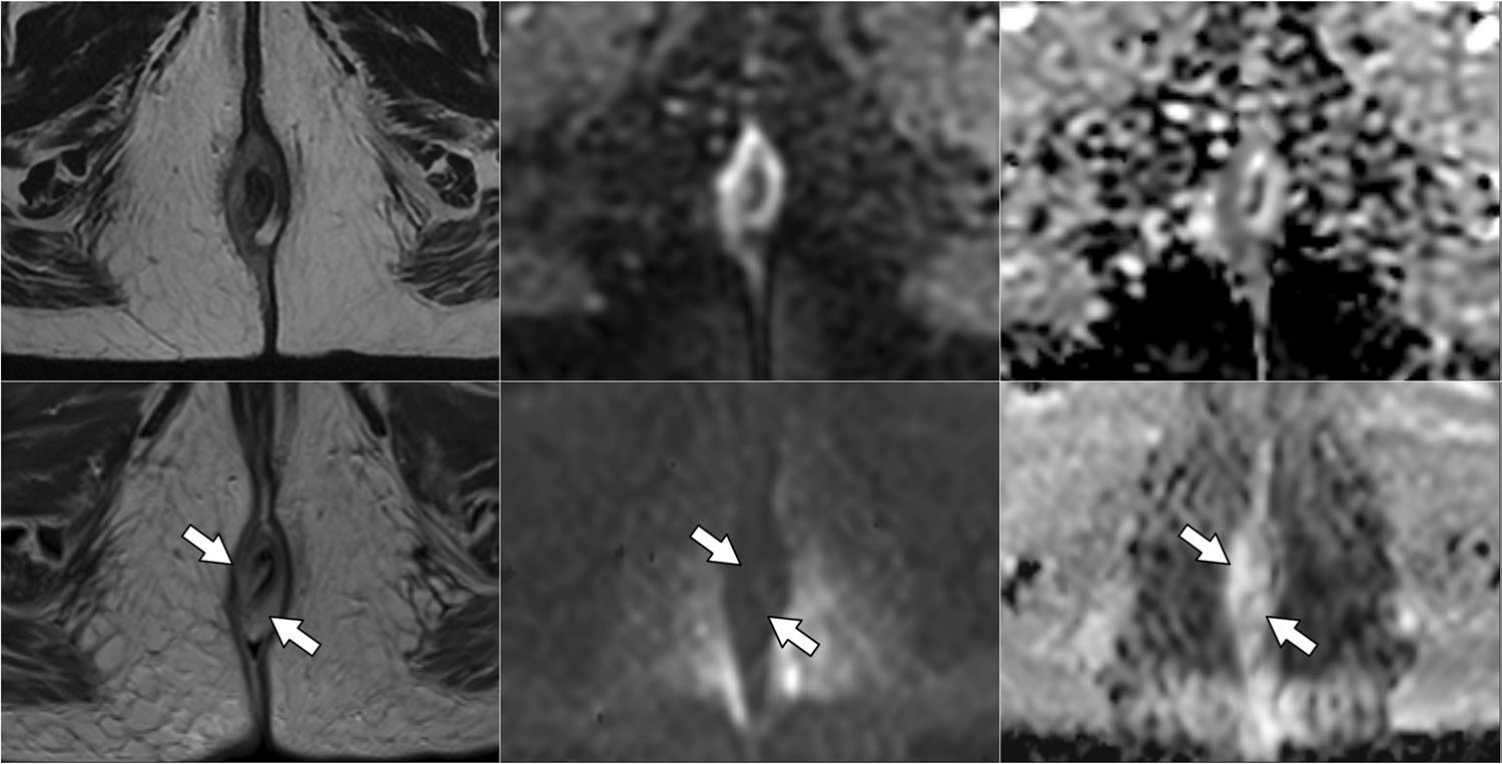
Images in a 53-year-old woman with squamous anal carcinoma. T2-weighted MRI (right column), high *b*-value DWI (middle column) and DWI ADC map (left column). At baseline MRI, tumour of the lower canal staged as T2 (upper row). Persistence of intermediate T2 signal (TRG-3) at the site of tumour (arrows) does not correspond to restricted diffusion, in keeping with excellent response (DWI-TRG-2)
